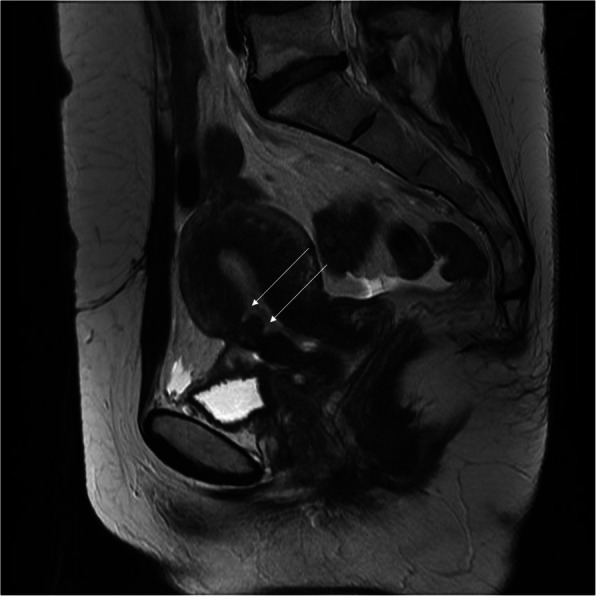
Pelvic MRI, FSE/T2WI in sagittal plane: two hyperintense niches after two C-sections (arrows)
